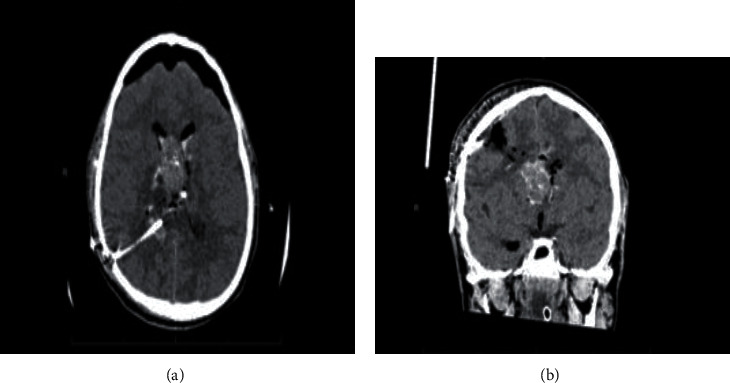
Head CT axial view post operatively.
